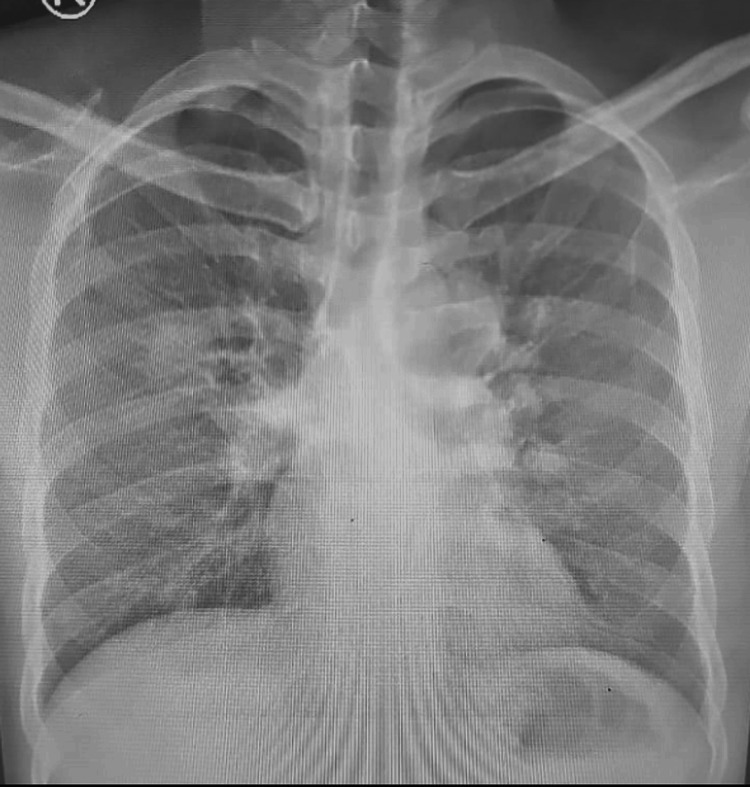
Chest X-ray on admission showing mediastinal widening suspicious for mediastinal masses, with no definite pneumonic consolidation
